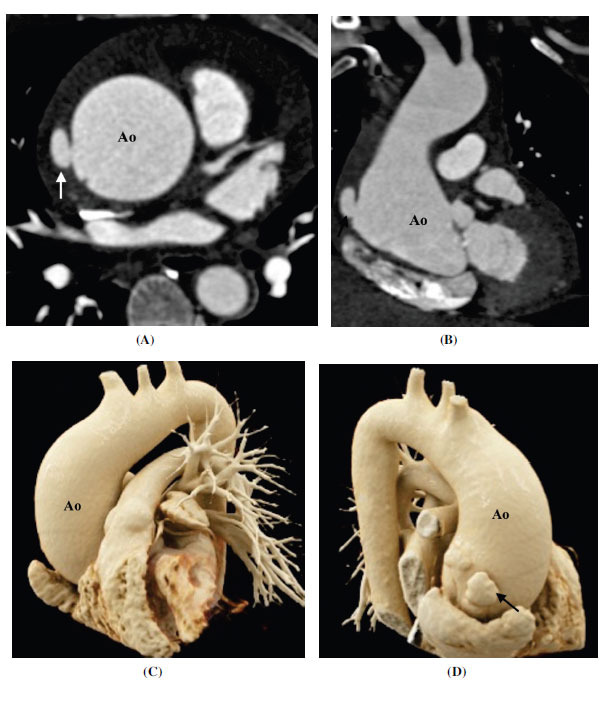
Type 2 bicuspid aortopathy. (A) Axial and (B) coronal contrast-enhanced computed tomographic angiography image showing dilated ascending aorta. A small outpouching suggesting a pseudoaneurysm is seen arising from the right lateral aortic wall. (C) Frontal and (D) back cinematic rendered image showing dilated ascending aorta and pseudoaneurysm. Abbreviation: Ao; ascending aorta.
